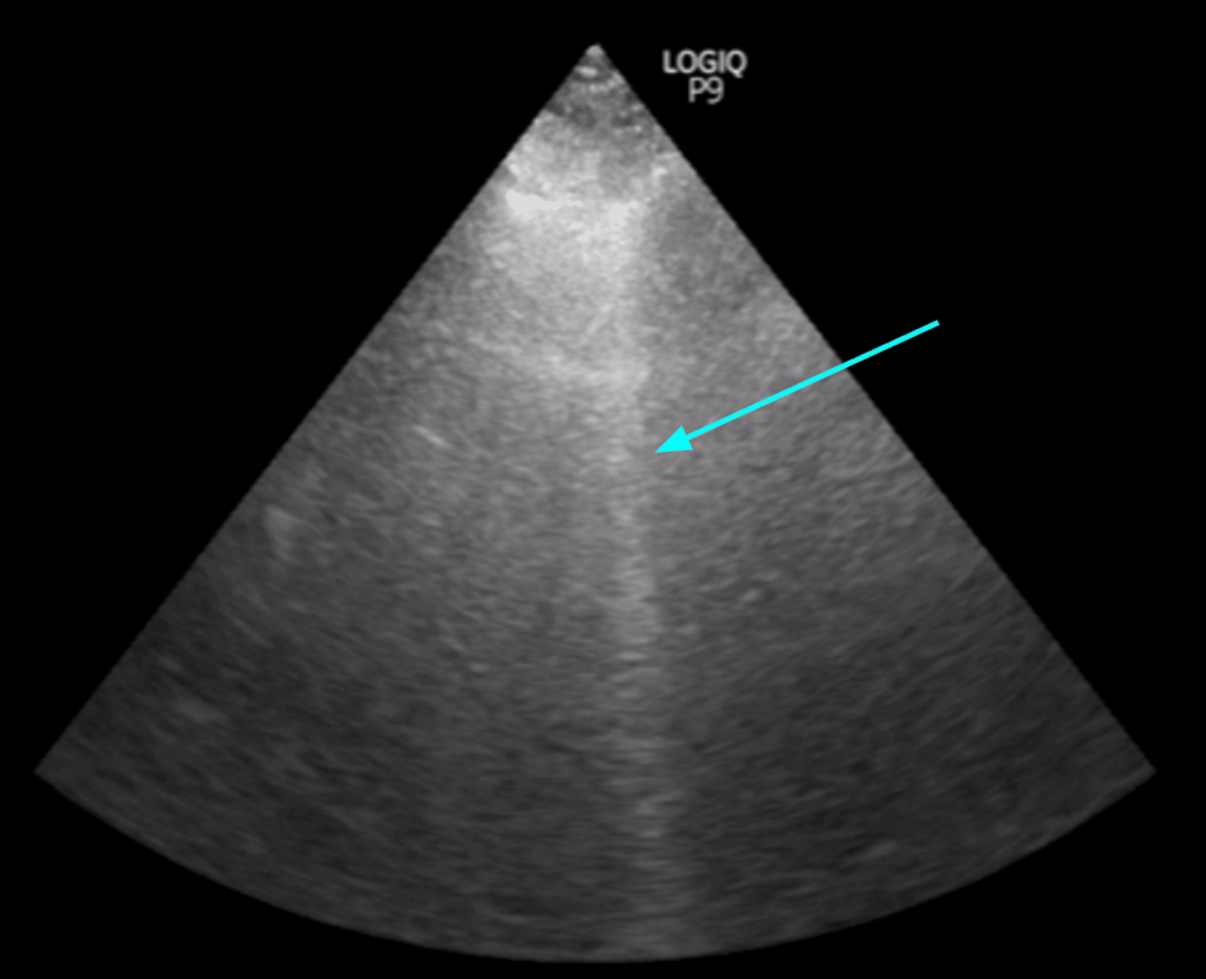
Chest ultrasonography showing prominent B-line (teal arrow) without pleural effusion at the right lung base.
